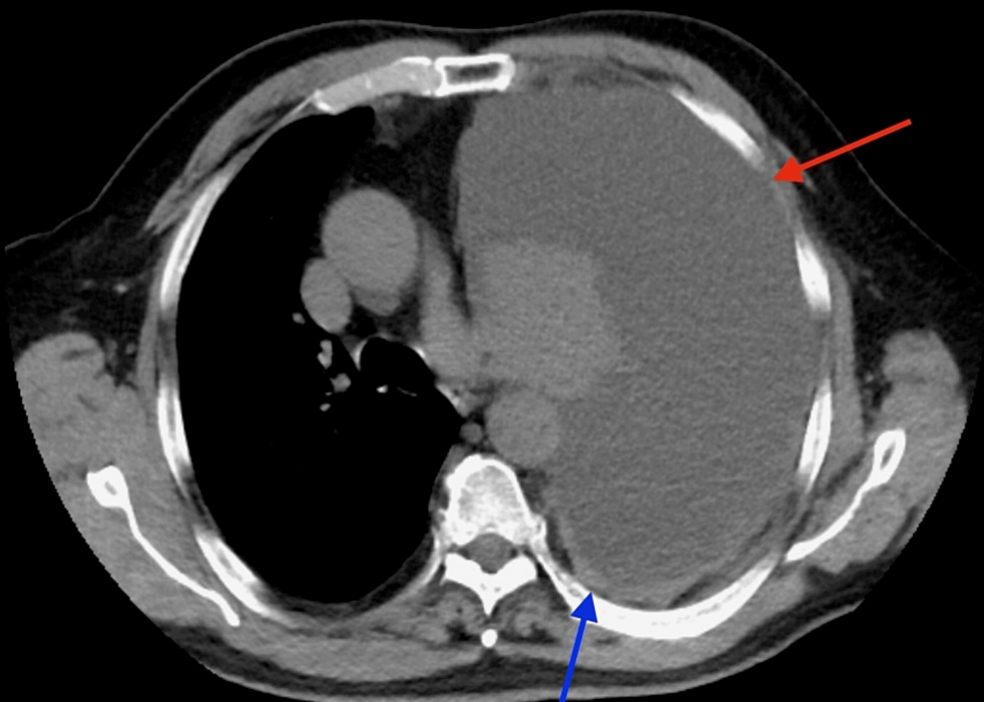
CT chest without contrast showing a large left-side pleural effusion (red arrow) with possible underlying pleural-based masses (blue arrow).
